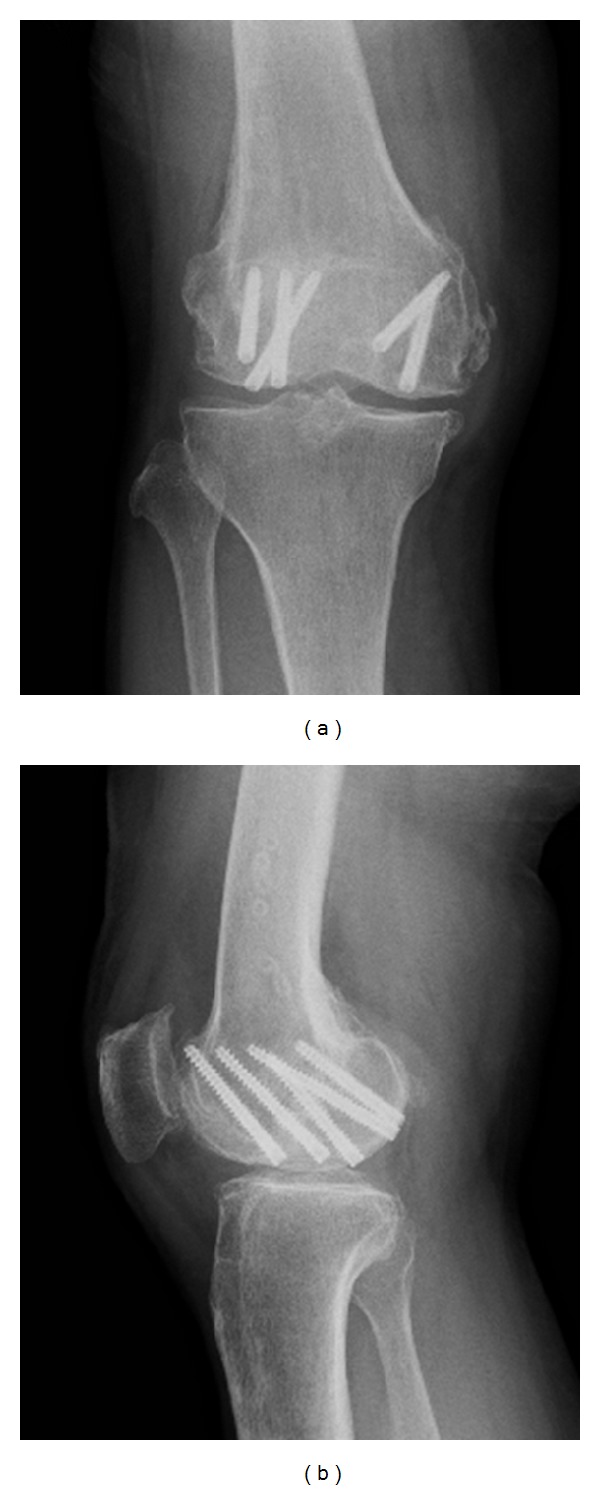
Anteroposterior (a) and lateral (b) radiographs obtained at the latest follow-up, at 2 years and 7 months after surgery.
